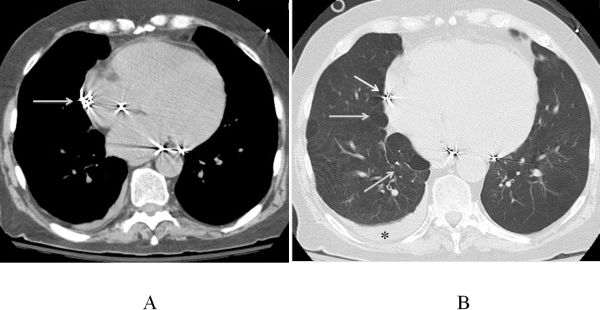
CT Scans of the chest (A) tip of the helix of the atrial lead  protruding just outside the cardiac  silhouette(Arrow). 2B: Centrilobular emphysema with bullous lesions near the lead tip and helix  shown (arrows). There is a small pleural effusion also seen (*)
